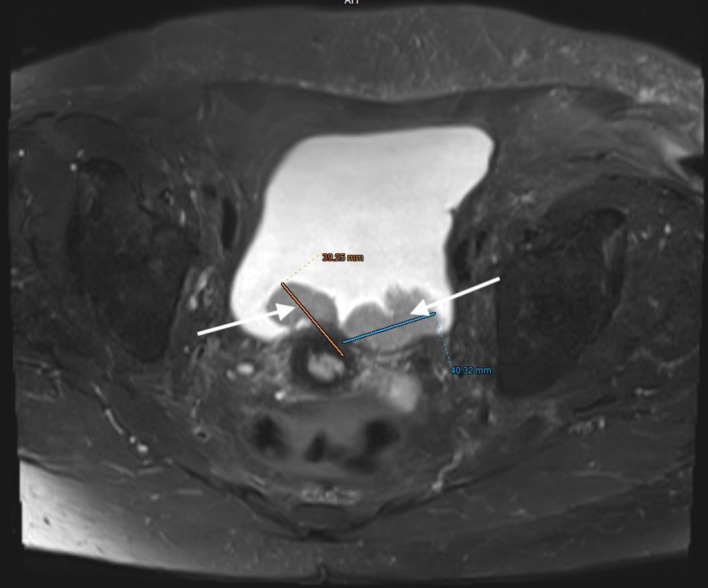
Lower abdominal magnetic resonance imaging (MRI) revealed a vegetative, infiltrative lesion invading the surrounding adipose tissue and occupying the urinary bladder cavity (arrows).
